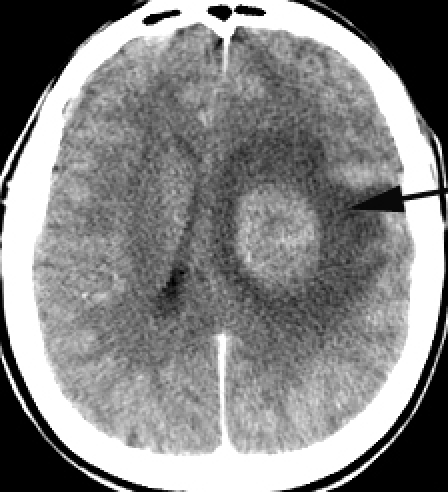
A contrast CT image of a deep frontal tumor with brain edema as a case of parenchymatous intracranial hypertension
